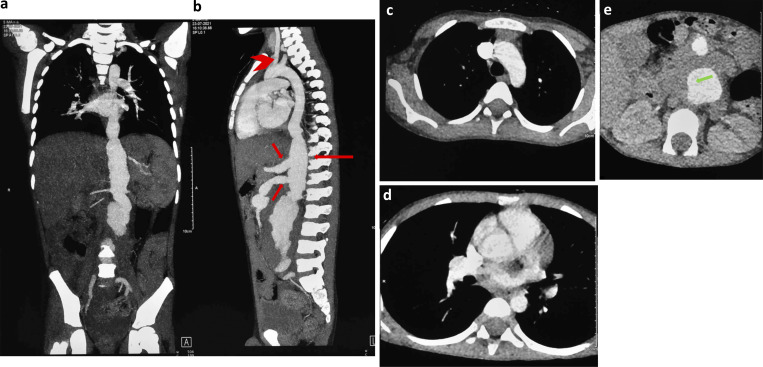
Panel of CT angiography images demonstrating vascular involvement of thoracic and abdominal vessels in Patient 2. (a–e) CT angiography of the thorax and abdomen of patient 2: coronal (a), sagittal (b), and axial (c–e) sections demonstrating the fusiform and irregular outline of the abdominal aorta (long red arrow in b) extending from the diaphragmatic hiatus to its bifurcation at the L5 vertebral level with a maximum transverse caliber of 2.7 cm. A thick rim of hypodense soft tissue is seen surrounding the aneurysmal segment suggestive of partial thrombosis (green arrow mark). Variable lengths of proximal parts of the celiac trunk (upper short red arrow in b), superior mesenteric artery (lower short red arrow in b), and left renal artery show fusiform dilatation. Aortic root, ascending aorta, aortic arch (c) and its branches (arrowhead), and descending thoracic aorta are normal in course, caliber, and contrast opacification. The two renal arteries are seen on the right side, and the inferior mesenteric artery is normal in course, caliber, and outline.
